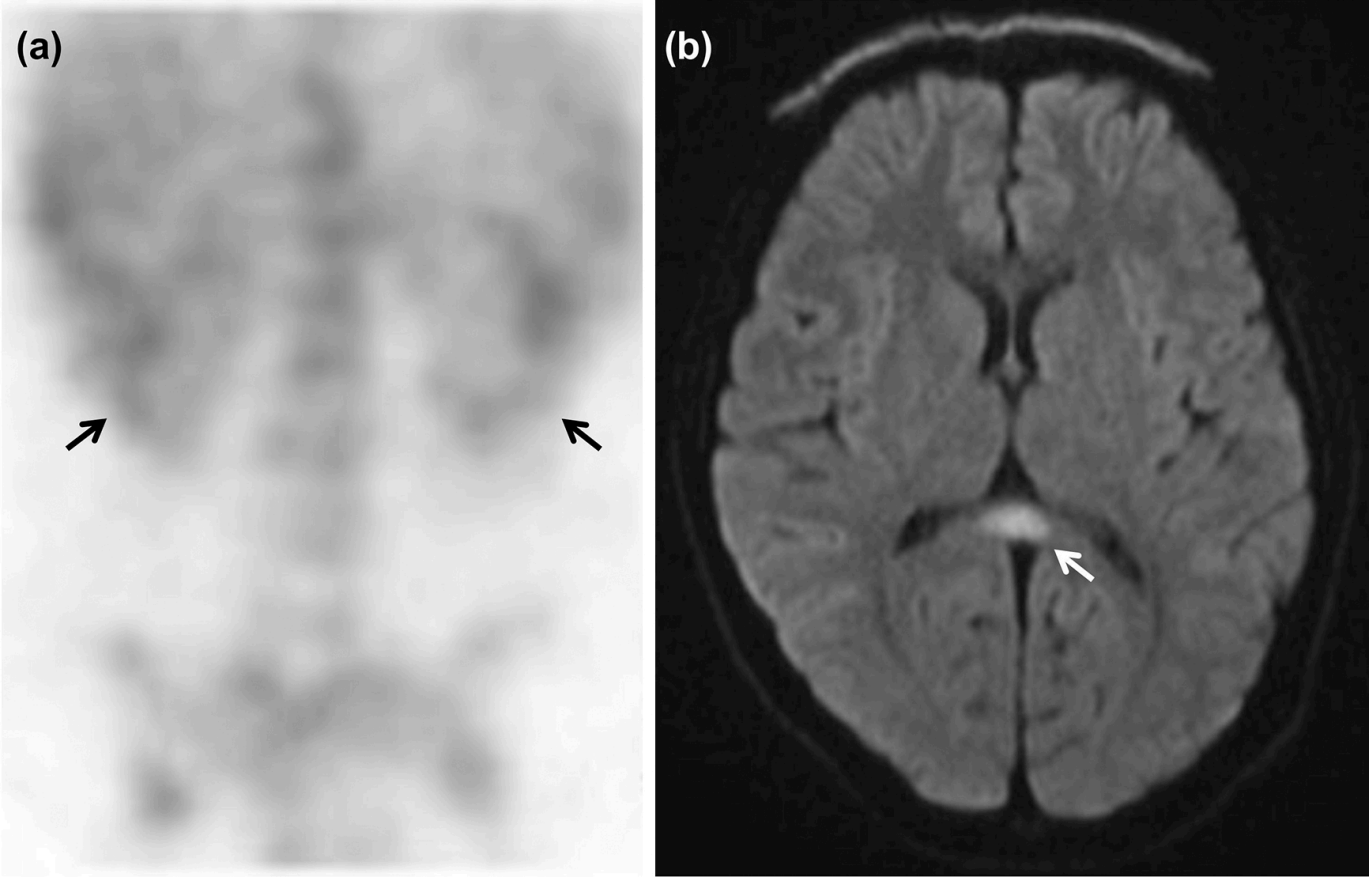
Results of 67Ga-citrate scintigraphy and brain magnetic resonance imaging (MRI). (a) The result of 67Ga-citrate scintigraphy indicates mild bilateral renal uptake (black arrows). (b) Axial MRI images. Diffusion-weighted images show a hyperintense area in the splenium of the corpus callosum (white arrow), which completely disappeared at the follow-up MRI.
